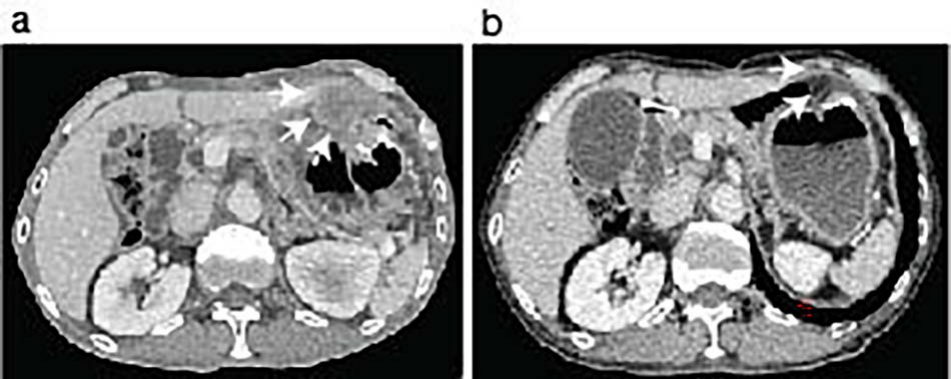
ICI therapy after the abdominal surgery. The patient showed peritoneal nodules (arrows) in the first follow-up CT scan after the last abdominal surgery (a). The peritoneal nodules were almost disappeared after 6 months following Pembrolizumab treatment (b, arrows).
